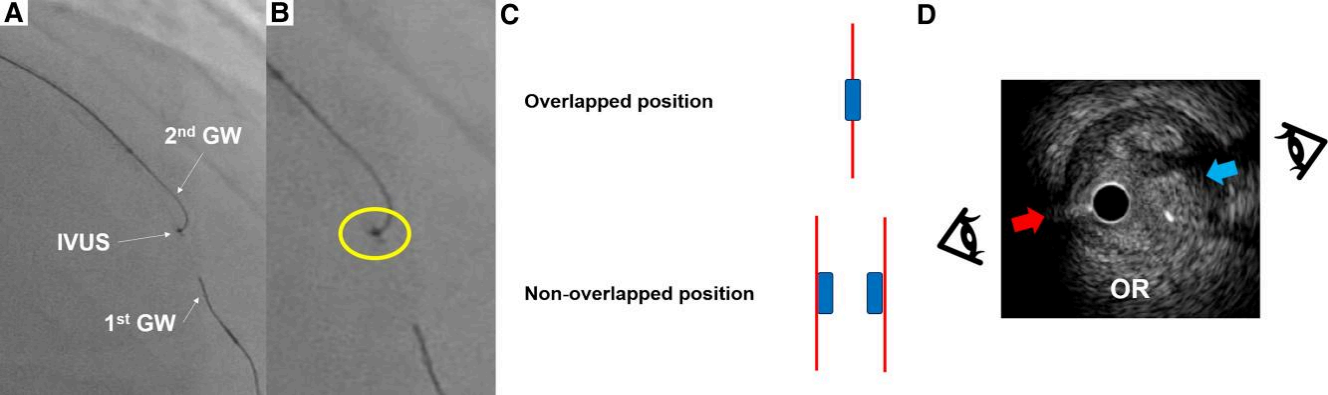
Identifying the fluoroscopic viewing direction based on the positional relationship between the first guidewire and IVUS catheter. (*A* and *B*) The first guidewire and intravascular ultrasound (IVUS) catheter appears overlapped from the right cranial fluoroscopic view. Also, the tip of the second guidewire looks like downward direction. (*C*) The relationship between the IVUS catheter and the first guidewire is classified into overlapped and non-overlapped positions based on the IVUS transducer (box) and the first guidewire (line). (*D*) The fluoroscopic viewing direction is from either 2 or 8 o’clock according to the positional relationship between the first guidewire and IVUS catheter.
